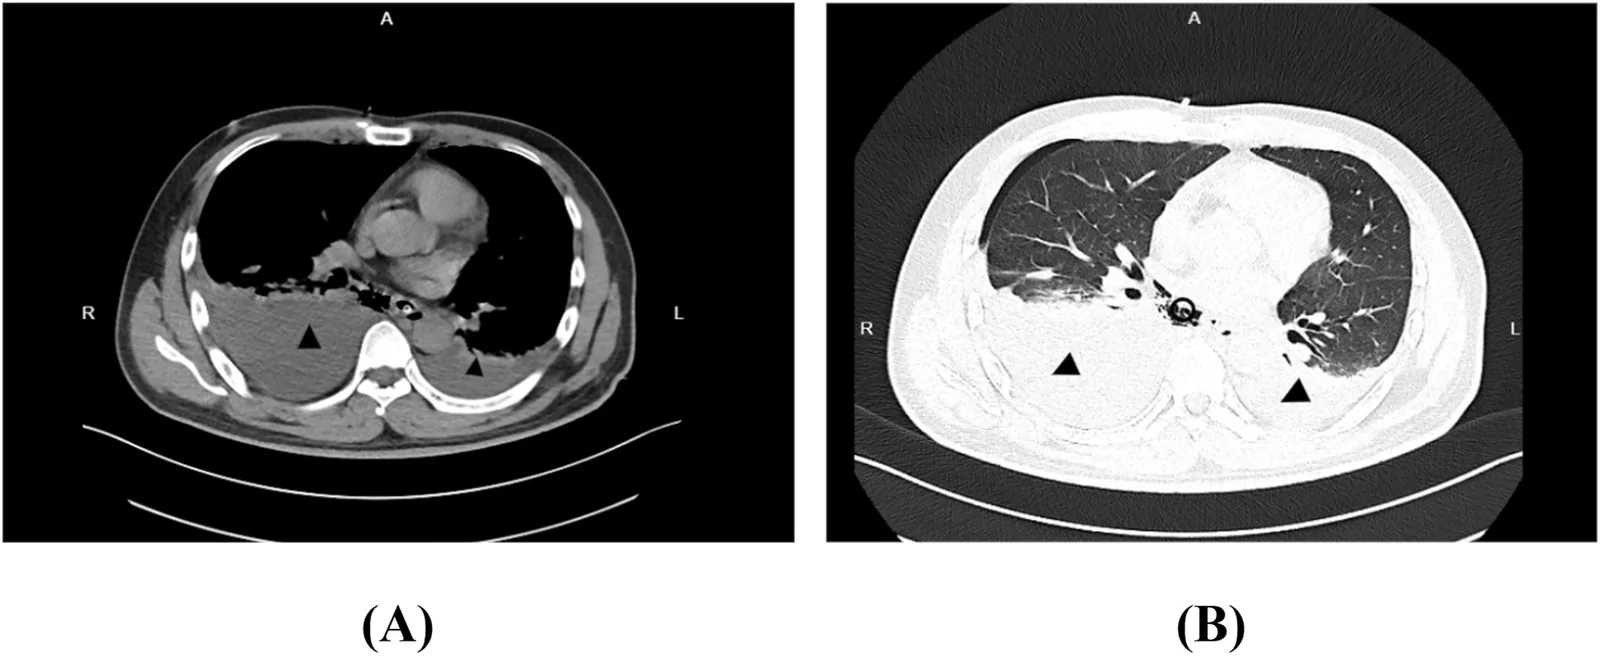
Initial radiological findings in boerhaave syndrome. (A) Contrast-enhanced chest CT demonstrating bilateral pleural effusions (▴). (B) Lung-window CT image showing bilateral pleural effusions with associated atelectasis (▴) and pneumomediastinum (○).
